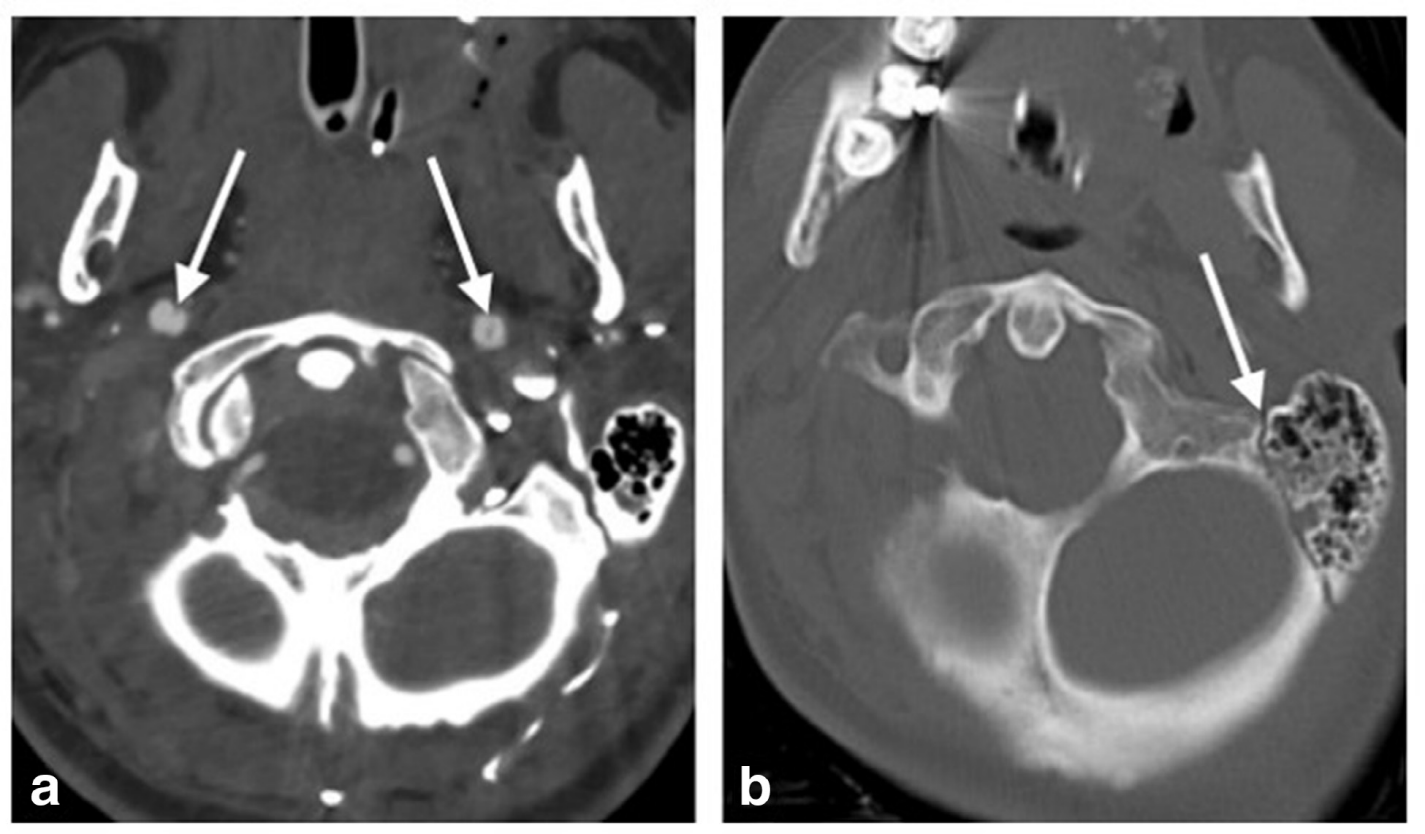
50-year-old female involved in accident whilst riding her motorcycle. a. Axial CTA image demonstrating bilateral internal carotid artery dissections at the C1 level (arrows). b. Axial image in bone windows demonstrate a left base of skull fracture involving the left temporal bone (arrow).
